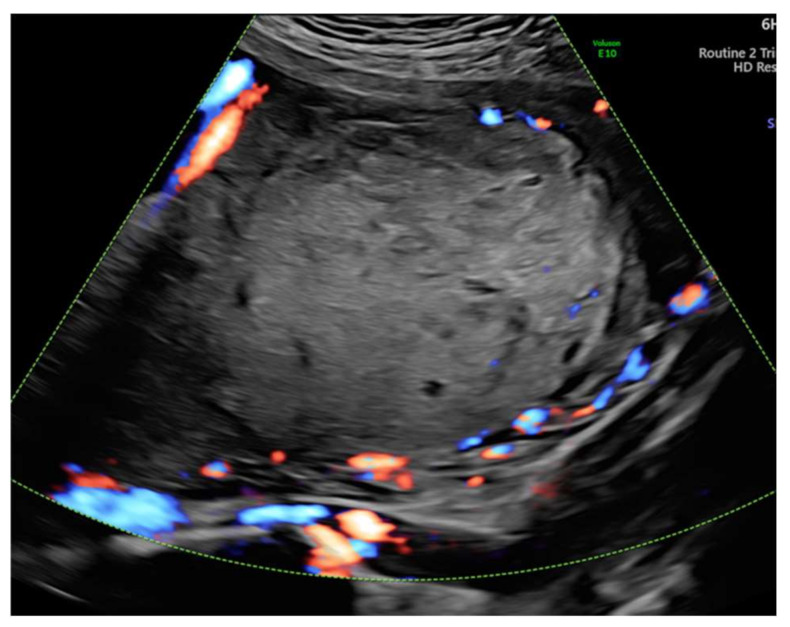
Doppler showed normal vascularization at 30 weeks of gestation.
